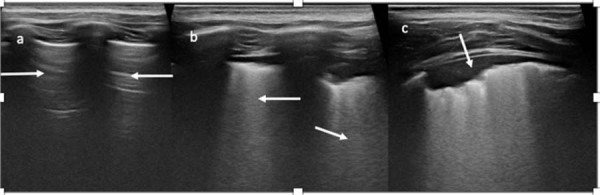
Achados representativos da ultrassonografia pulmonar no edema pulmonar. a) Linhas A dominantes na zona superior do pulmão esquerdo (setas). (b) Linhas B confluentes na zona média do pulmão esquerdo (setas). (c) Pequena consolidação periférica na zona inferior do pulmão direito (seta) associada a linhas B que se misturam.
